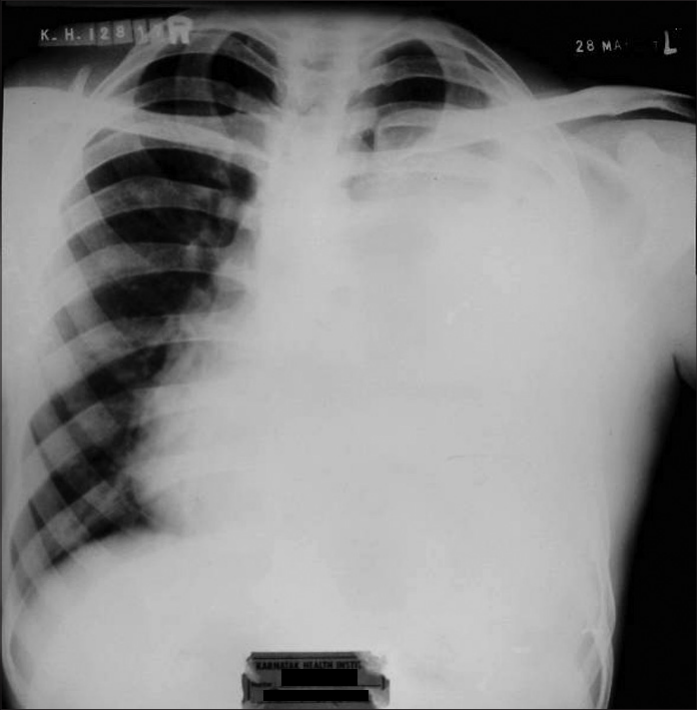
Chest radiograph showing large homogenous density occupying left hemi-thorax with obliteration of the left heart border and mediastinal shift to the right side
